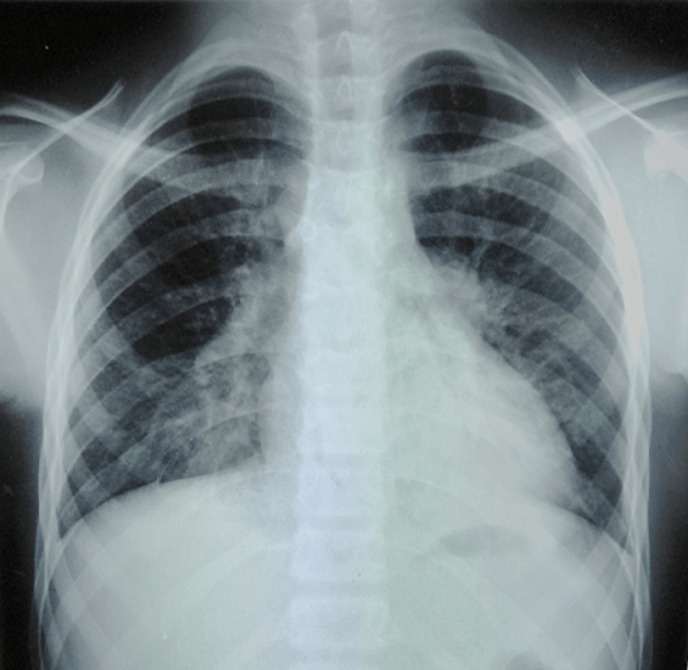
chest X-ray showed normal size heart with bilateral base-predominant alveolar and interstitial infiltrates
